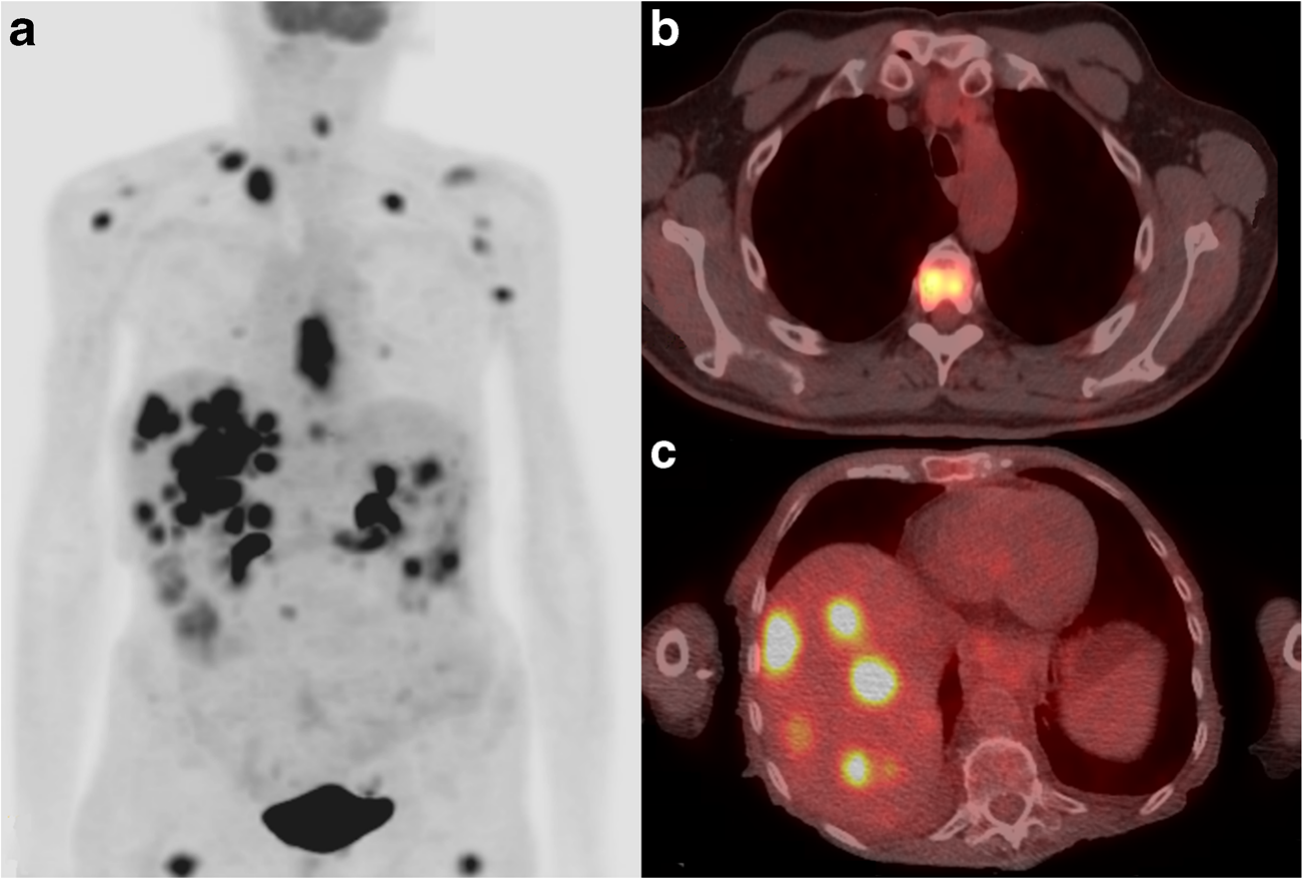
Examples of true positive metastatic lesions detected by ^18^F-FDG PET/CT restaging. (a/c): 80-year-old woman with adenocarcinoma of the distal esophagus treated with chemoradiation. The maximum intensity projection PET image shows multiple hypermetabolic foci of the liver and multiple soft tissue lesions that were confirmed malignant with follow-up scans. (b): 65-year-old male with squamous cell carcinoma of the distal esophagus who had undergone chemoradiotherapy. The PET/CT image showed ^18^F-FDG accumulation in the liver and in the thoracic spine at T5. Follow-up CT showed disease progression
